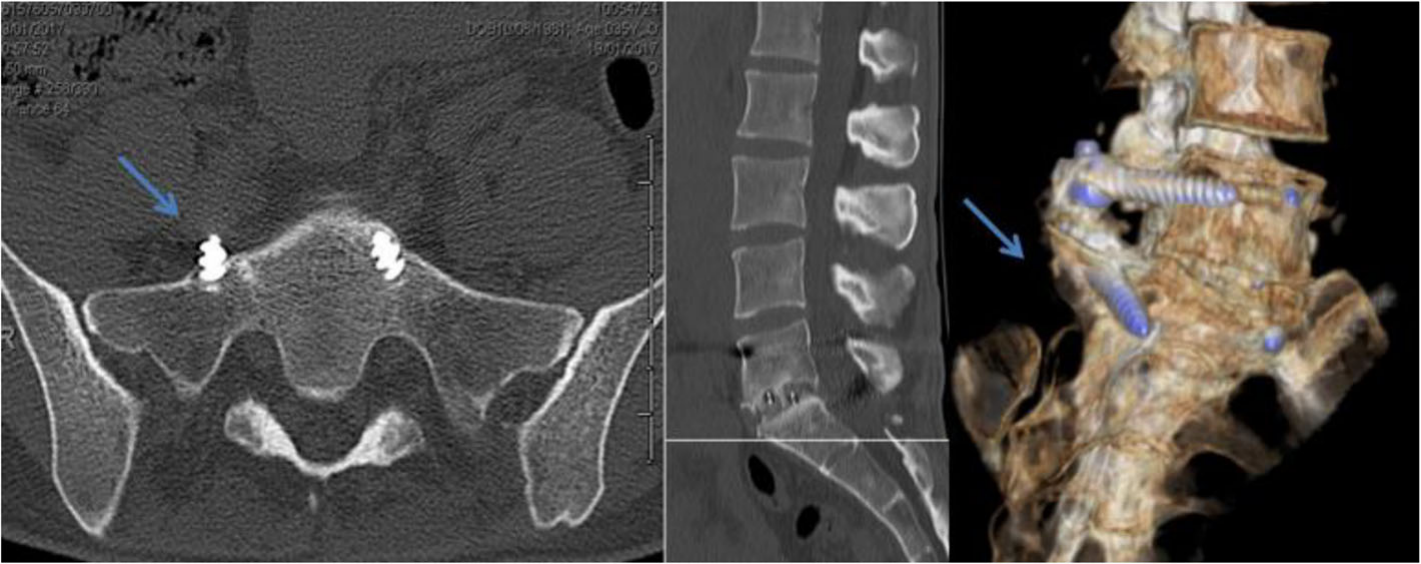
Lumbar CT scan. L5-S1 Fusion complication, anterior cortical perforation of vertebral body. S1 right side (blue arrows)
